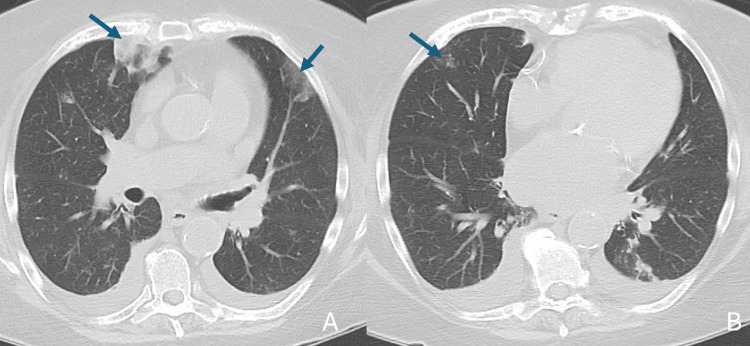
CT of chest showing consolidation and ground-glass opacities (arrows)
